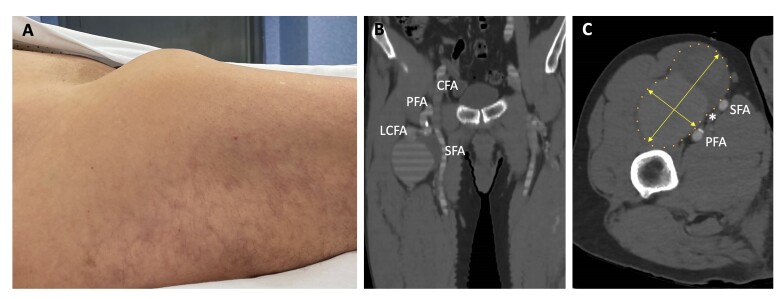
(A) Palpable pulsatile mass inferior to the right inguinal ligament; (B) CTA: Aneurysm originated from the right lateral circumflex femoral artery; (C) CTA: Aneurysm sac size (90x50 mm) and common femoral vein compressed by the aneurysm (*). CTA: Computed tomography angiography; CFA: Common femoral artery; PFA: Profunda femoris artery; SFA: Superficial femoral artery; LCFA: Lateral circumflex femoral artery.
